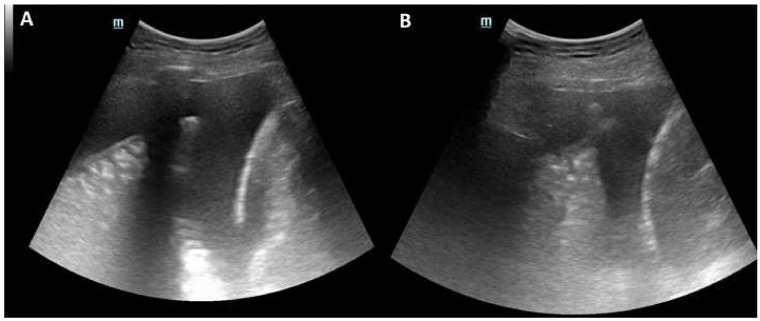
(A) Thoracic ultrasound (TUS) showed a moderate right hyperechogenic pleural fluid; (B) Atelectasis of right lower lobe.
